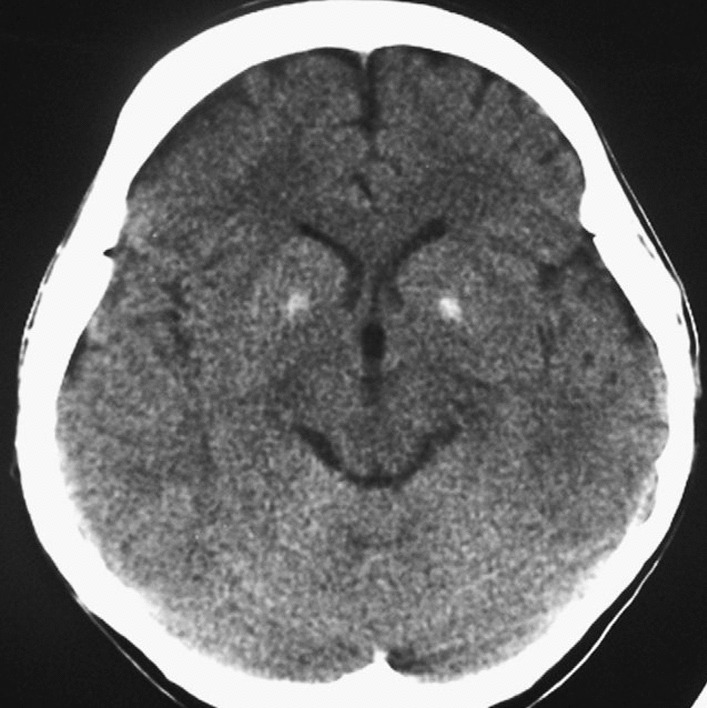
Showing evidence of basal ganglia calcifications in noncontrast computed tomography of the brain
